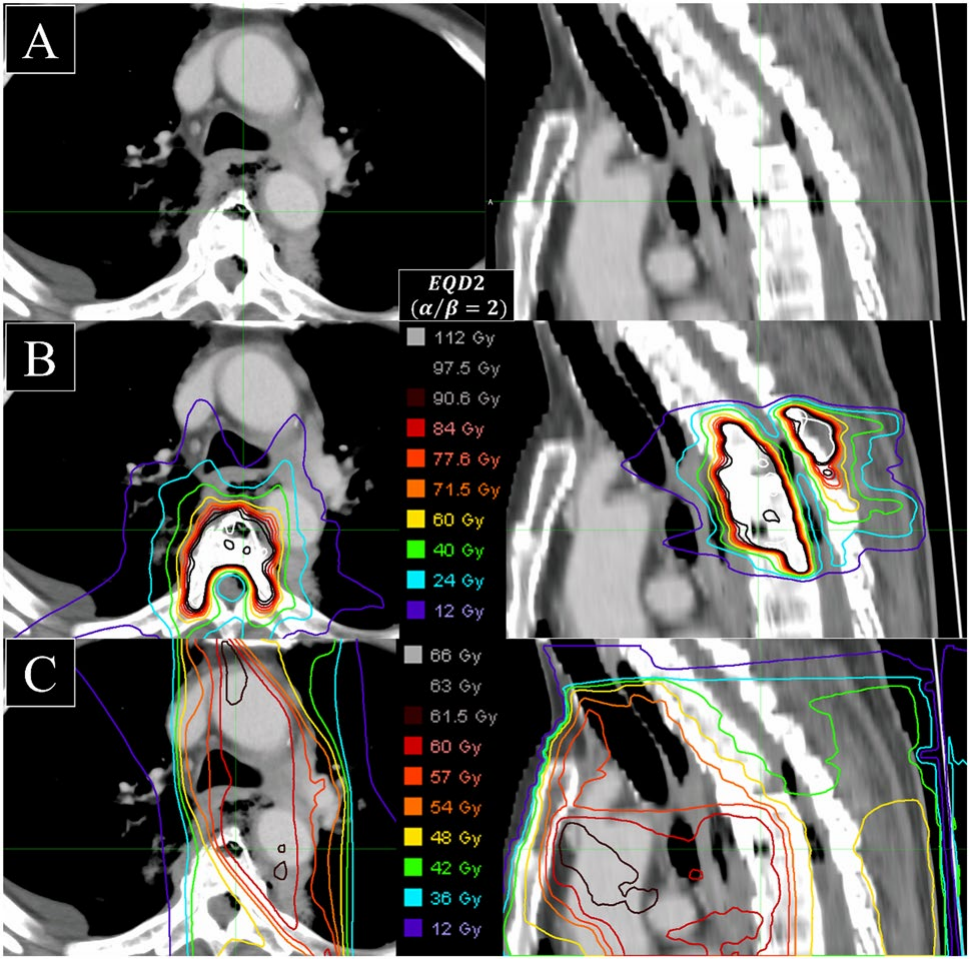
The images of diagnostic CT of radiation-induced esophageal perforation. A The diagnostic image. The patient was urgently hospitalized 18 days before death due to a fever and developed paraplegia 12 days before death. The image shows esophageal perforation, mediastinitis, and spinal cord compression due to an epidural abscess. B Dose distribution of re-irradiation SBRT on the fused-CT image (same CT as Fig. 2A). C Dose distribution of initial EBRT on the fused-CT image (same CT as Fig. 2A). *CT* computed tomography, *SBRT* stereotactic body radiation therapy, *EBRT* external beam radiation therapy
